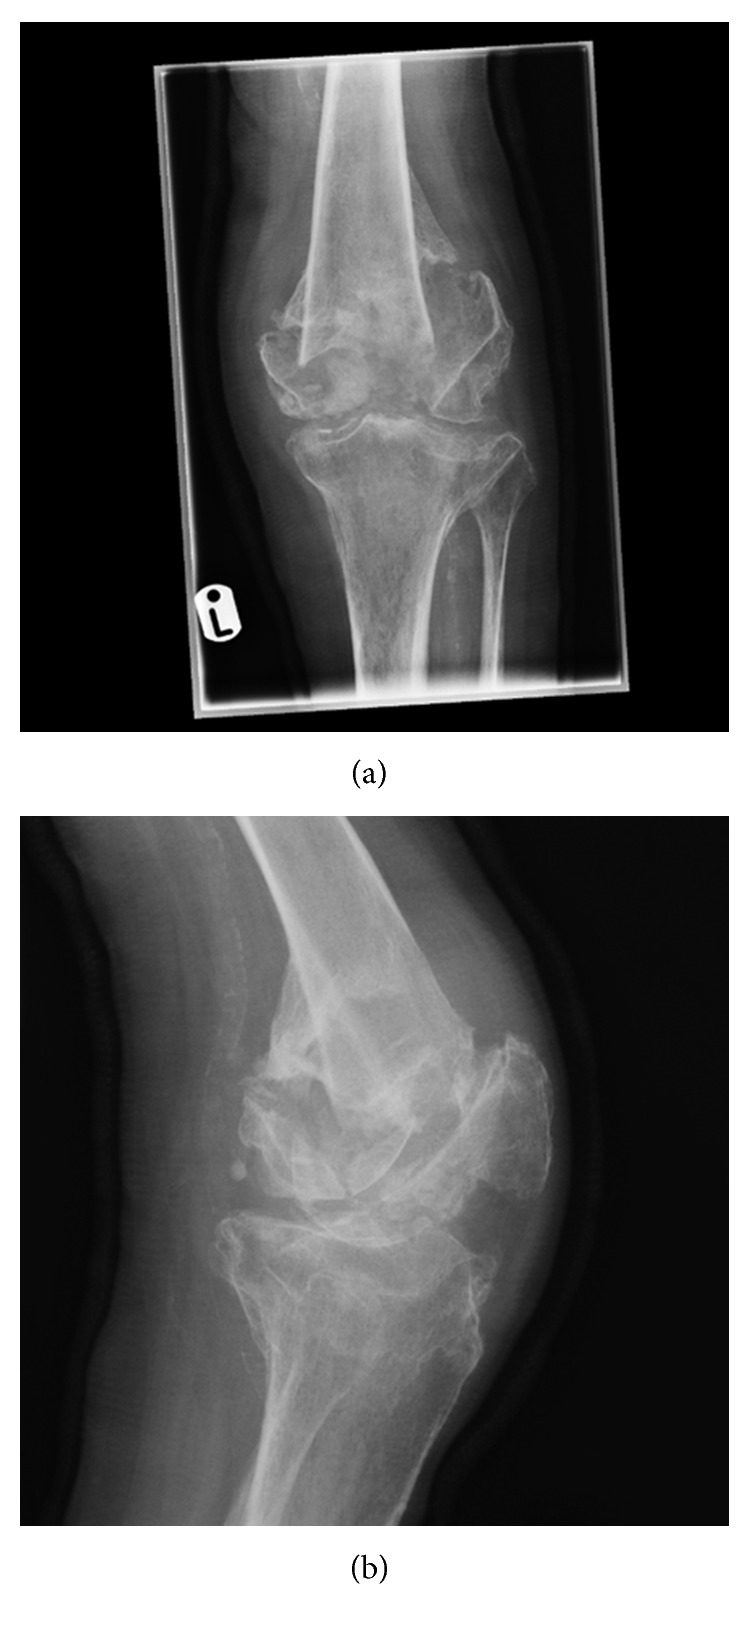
(a) AP and (b) lateral radiographs demonstrating ongoing nonunion and progressive deformity.
